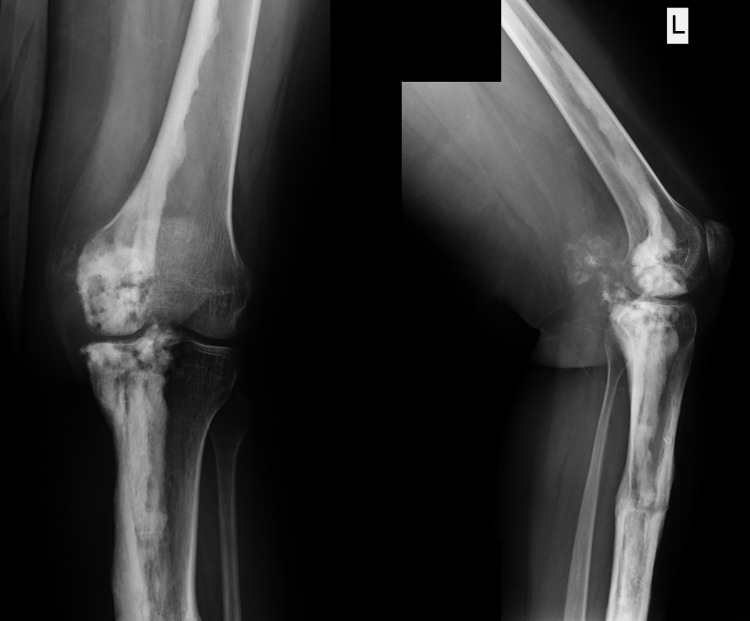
Plain radiographs of the left knee at 3-year follow-up. Although the deformity is corrected, the melorheostosis is still present.
